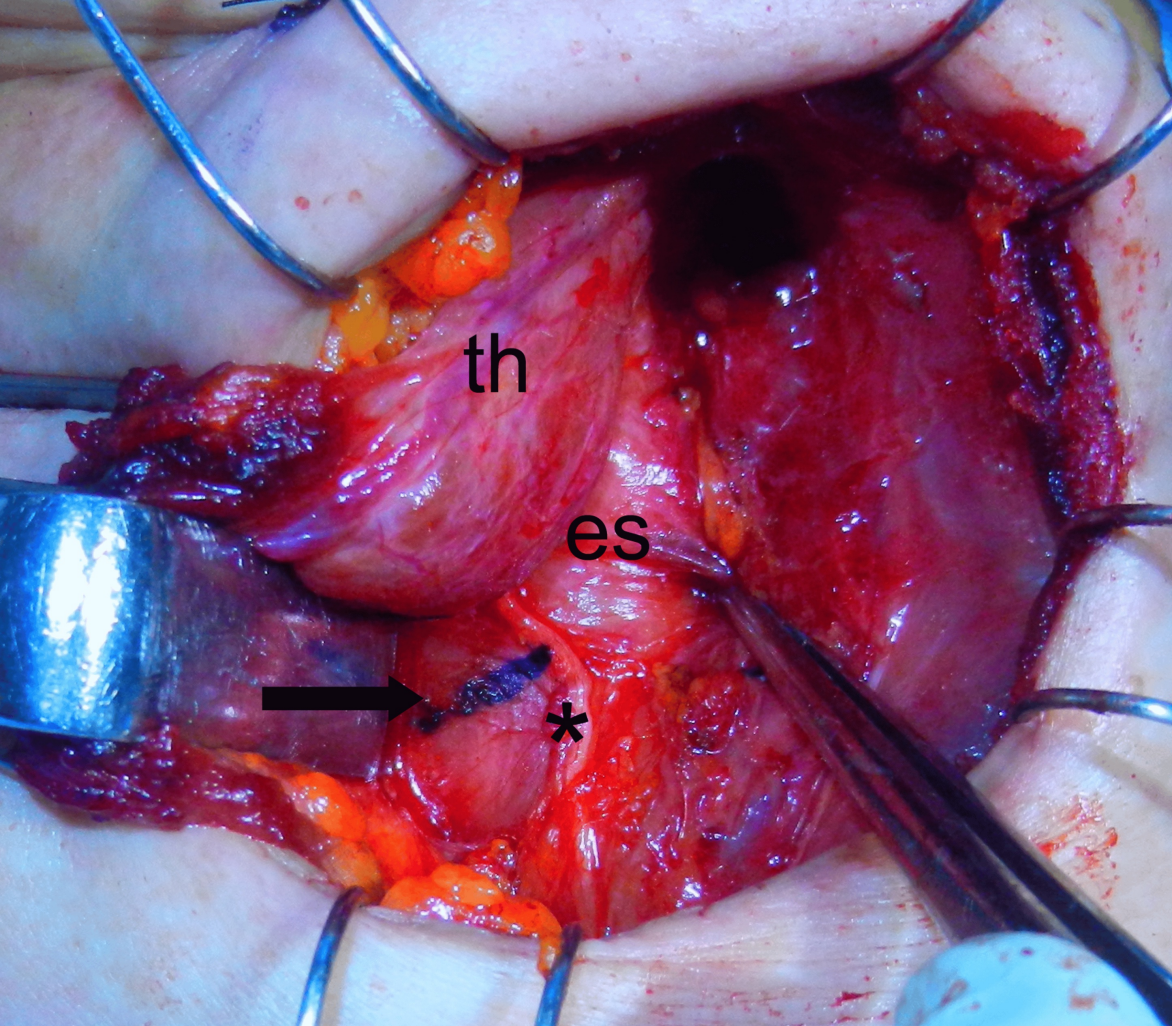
Intraoperative view of the esophageal surface in the neck. The incision site marked in blue (arrow) on the esophageal wall was confirmed by intraoperative ultrasonography. *, left recurrent neve; th, left lobe of the thyroid gland; es, esophagus
